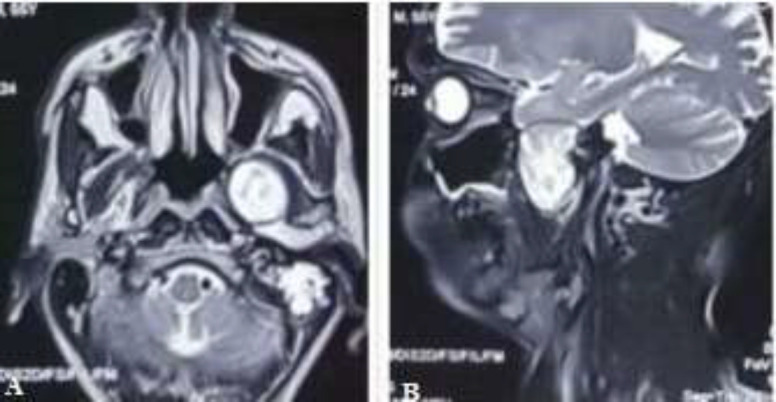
Contrast-enhanced MRI (T2- weighted) A; axial section revealed a heterogeneously enhancing mass in the infratemporal fossa, B; parasagittal section shows the extension of the tumor to the skull base
